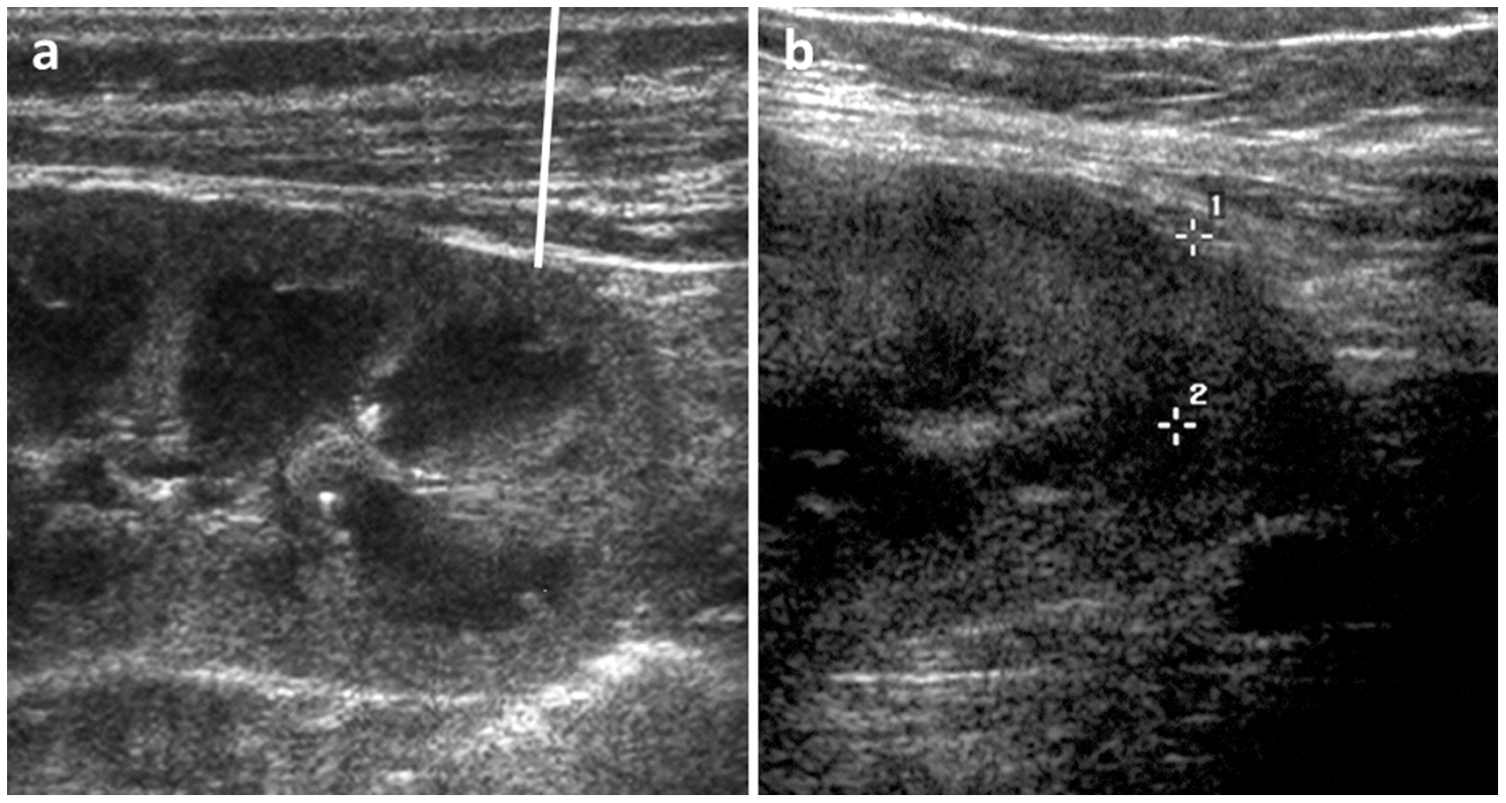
B mode sonographic image of a kidney. The distance between the lower kidney pole, the destined localization of biopsy and the skin was determined. Depth of biopsy was aligned on the measured skin-kidney pole distance (white line).
